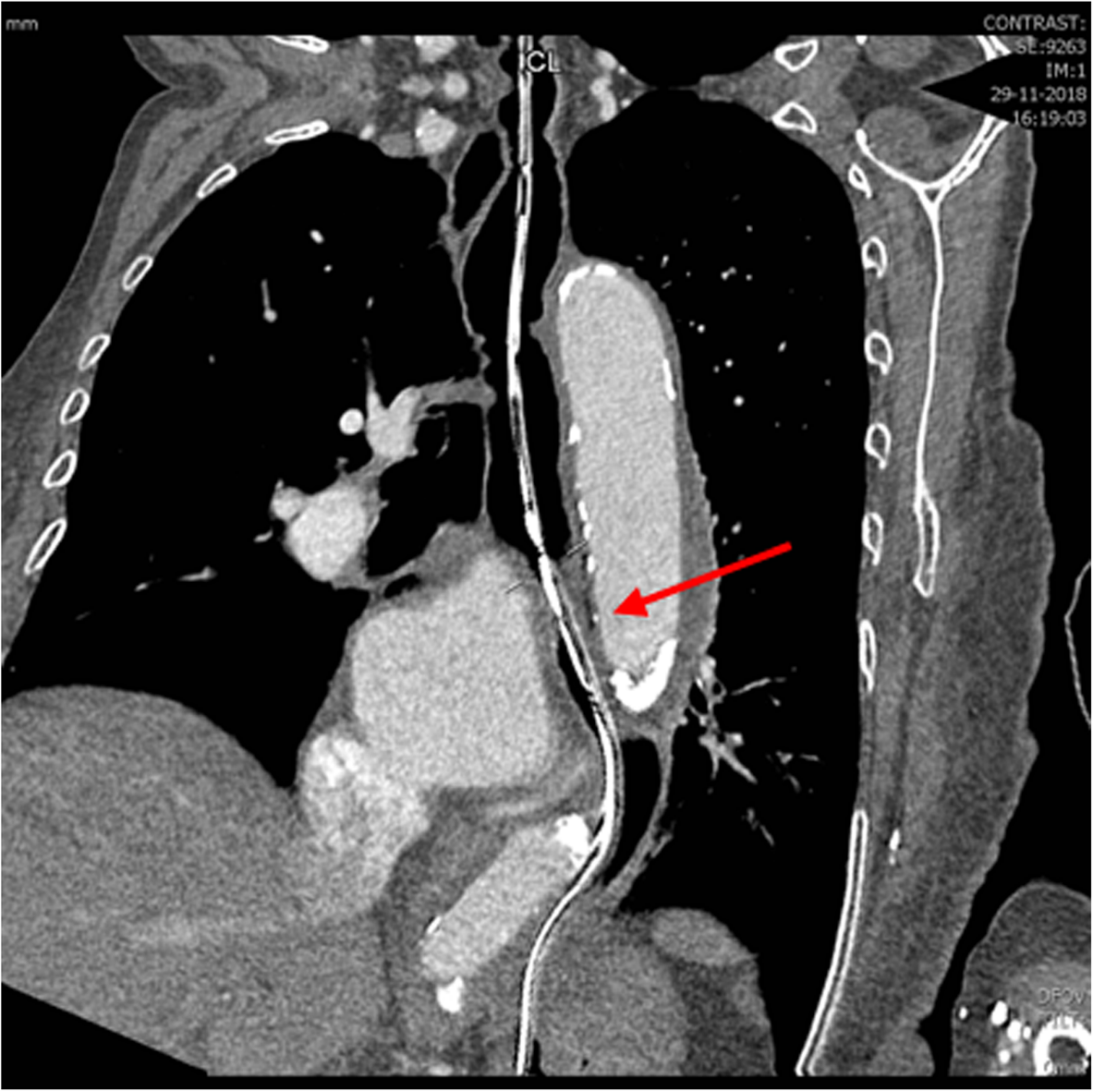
Coronal CT reconstructed image on esophagus; arrow indicates compression of distal esophagus (with nasogastric tube in situ) by aortic aneurysm
